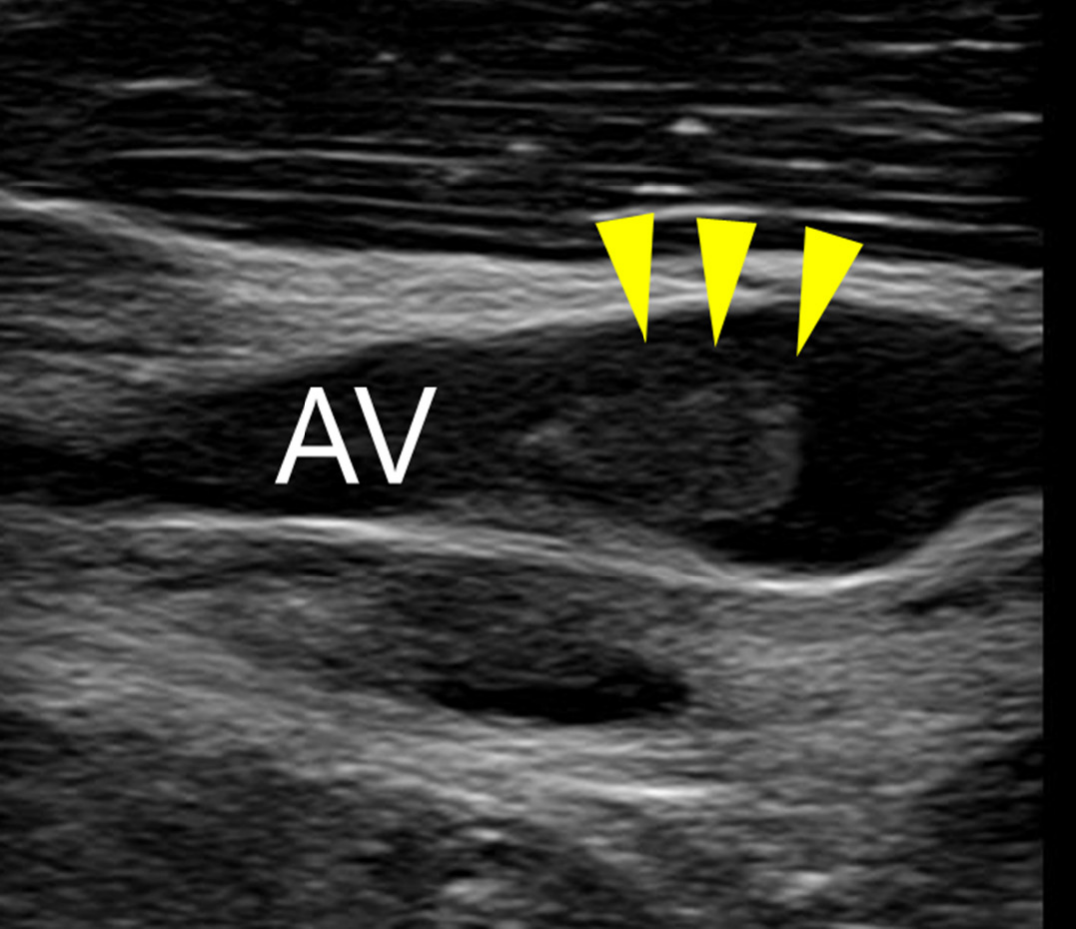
Venous ultrasound showed a 5 mm thrombus in the left axillary vein.
